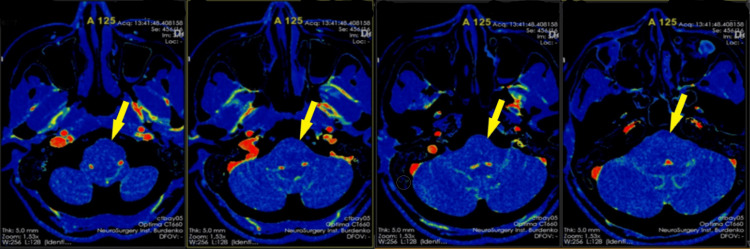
CT perfusion of the brain before endoscopic transnasal transclival tumor removal. CT axial plane images: tumor mass with low blood flow rates (indicated by the yellow arrow). CT, computed tomography
